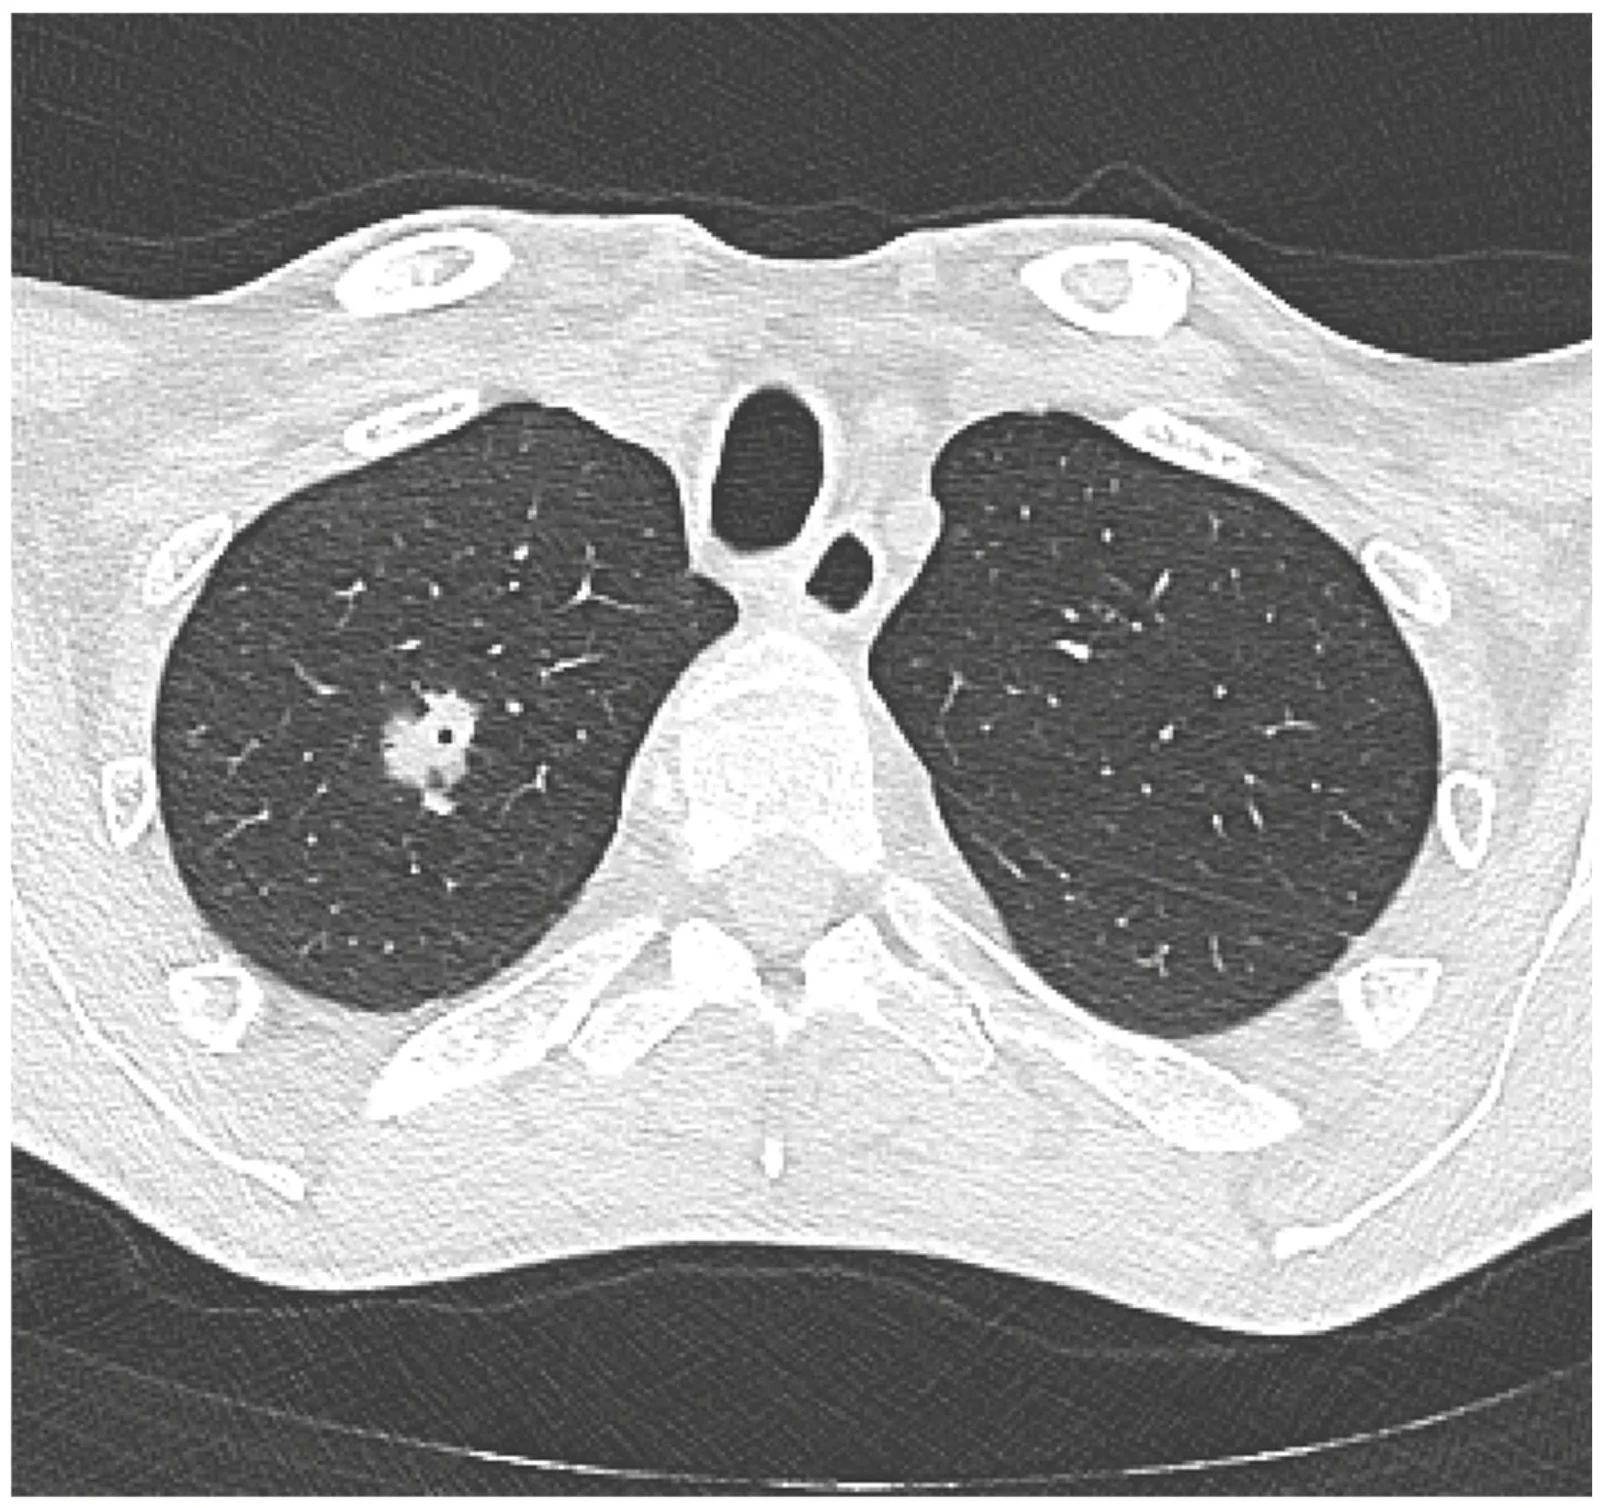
HRCT, axial plane. Primary pulmonary TB parenchymal lesion in the right upper lobe in an immunocompetent male patient. Initial cavitation is forming in the lesion.
